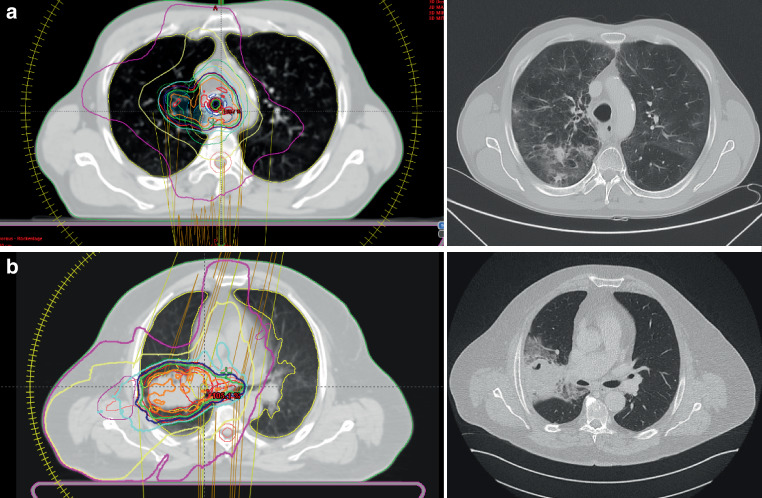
a Example of Immune-checkpoint inhibitor pneumonitis (ICI-P): Radiation-planning CT (left) and CT for pneumonitis evaluation (right). Bilateral Ground glass opacities with diffuse distribution and no sharp borders suggesting ICI-Pneumonitis. b Example of Radiation-induced pneumonitis: Radiation-planning CT (left) and CT for pneumonitis evaluation (right). Consolidation with sharp borders, unilateral and within the main areas of the radiation field
